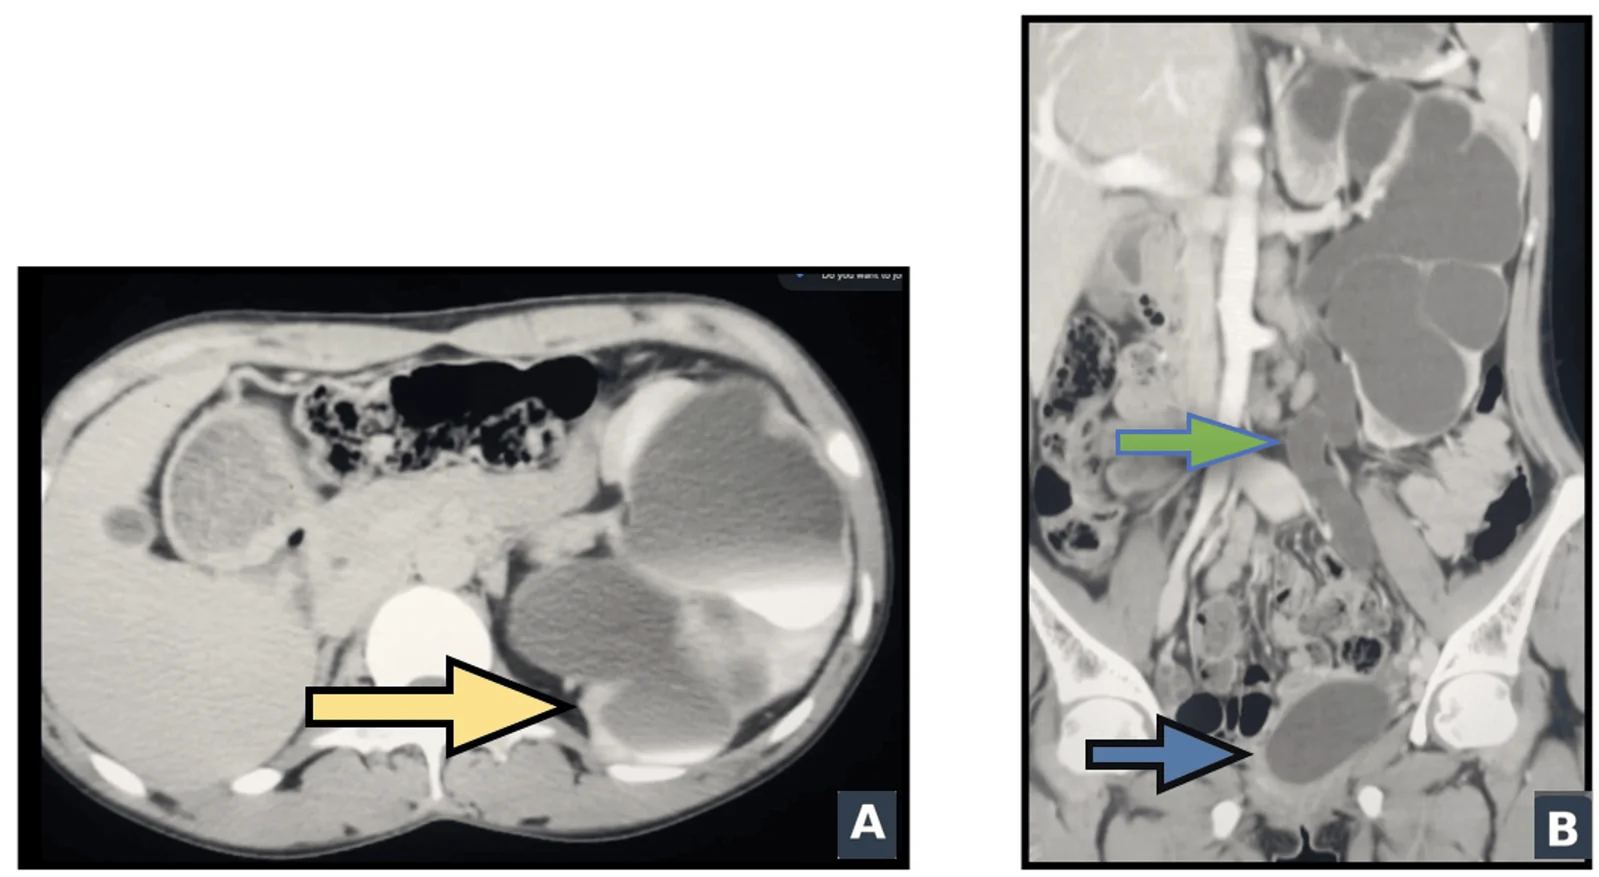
Preoperative computed tomography imaging: (A) axial section showing gross hydronephrosis with pooling of contrast on delayed phase (yellow arrow) and (B) coronal section showing dilated tortuous ureter (green arrow) and dilated lower ureter mimicking bladder (blue arrow) which was confirmed on retrograde pyelography
